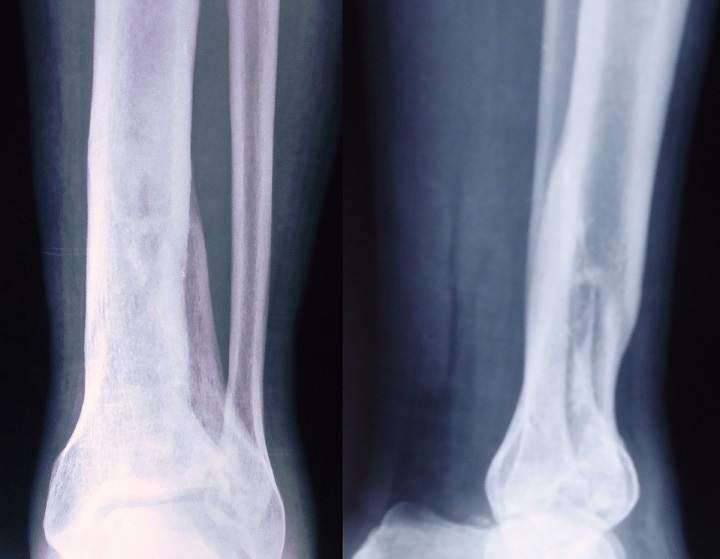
Follow-up radiographs after 42 months, showing good fracture union
